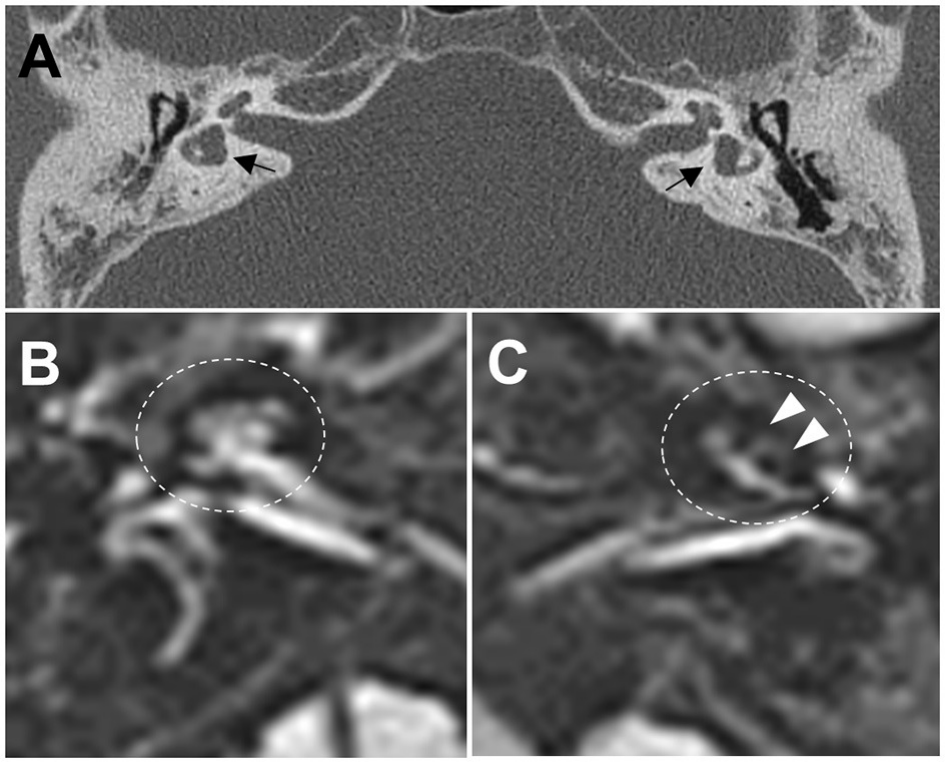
(A) Computed tomography images of the temporal bone demonstrate bilateral widening of the vestibules with an abnormally small bony island of lateral semicircular canals (black arrows) and soft tissue densities in the middle ear, and decreased mastoid air cells. (B,C) Axial three-dimensional fluid-attenuated inversion recovery images obtained 4 h after intravenous administration of gadolinium reveal cochlear endolymphatic hydrops (EH) with dilation of the scala media in the left ear (C, white arrows), but no EH in the right ear (B).
